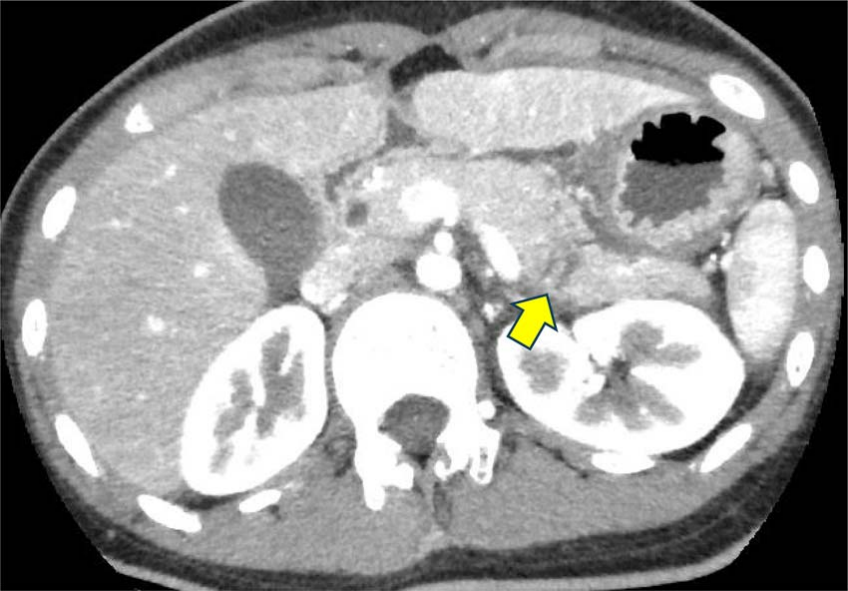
Contrast-enhanced CT on admission. Linear areas with poor contrast in the pancreatic body and increased peripancreatic fatty tissue density were observed.
